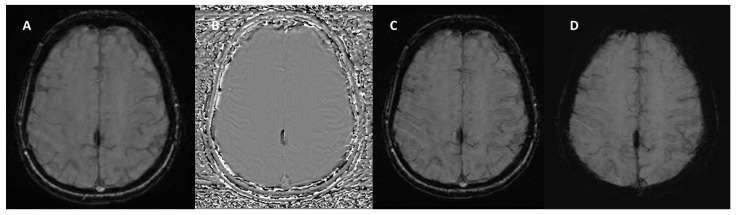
SWI sequence from the Siemens MAGNETOM Aera (Erlangen, Germany) 1.5T MRI scanner in a patient with calcification of the falx cerebri. (A) Magnitude Image: displays the calcification as a hypointense structure. (B) Phase Image: the calcification appears hypointense, consistent with its diamagnetic properties. (C) SWI-Processed Image and (D) Minimum Intensity Projection enhance the contrast of calcified structures and highlight low-signal calcification.
